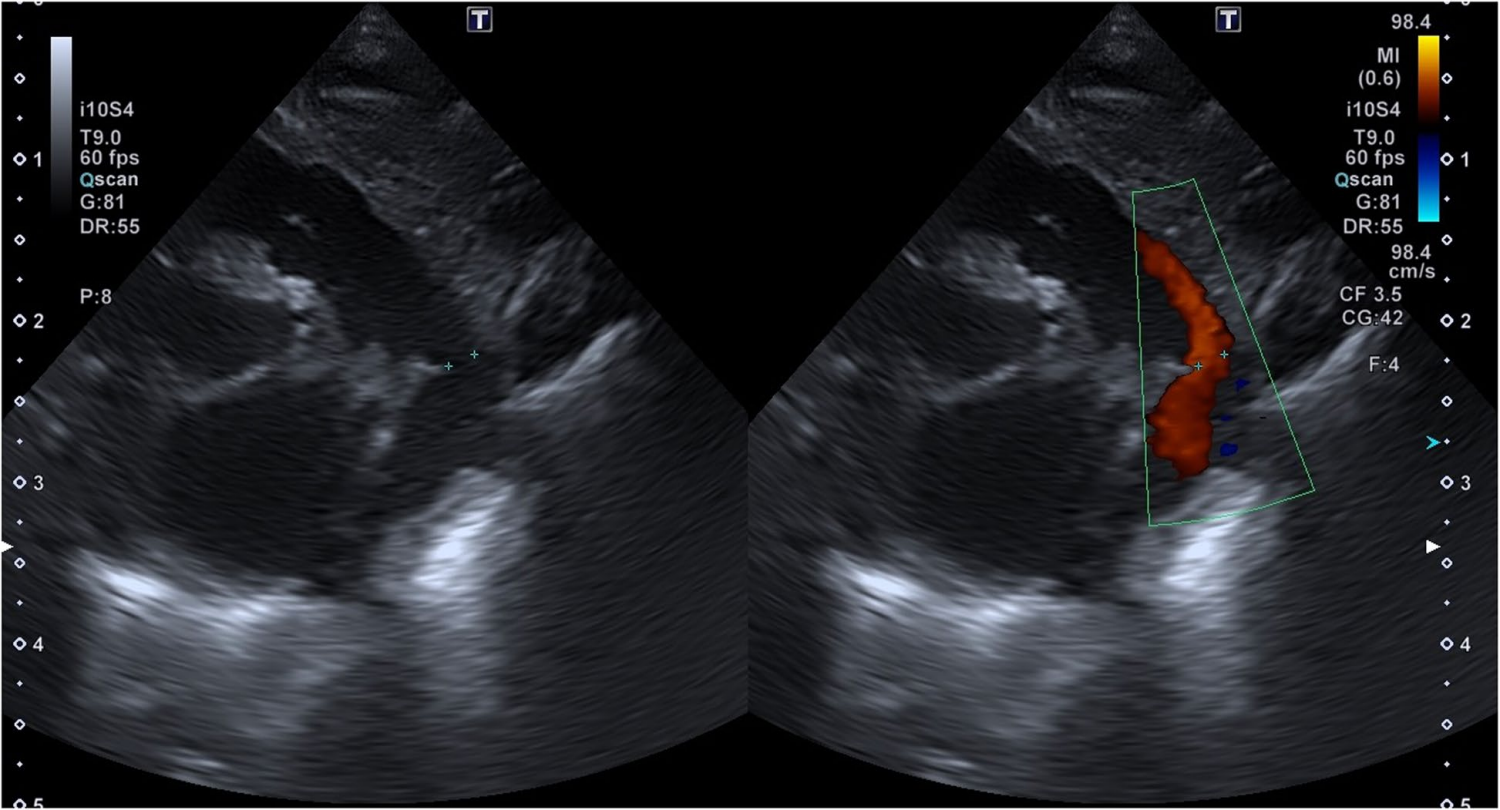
Echocardiographic measurement of the patent duct ‘s diameter in a short axis scan
